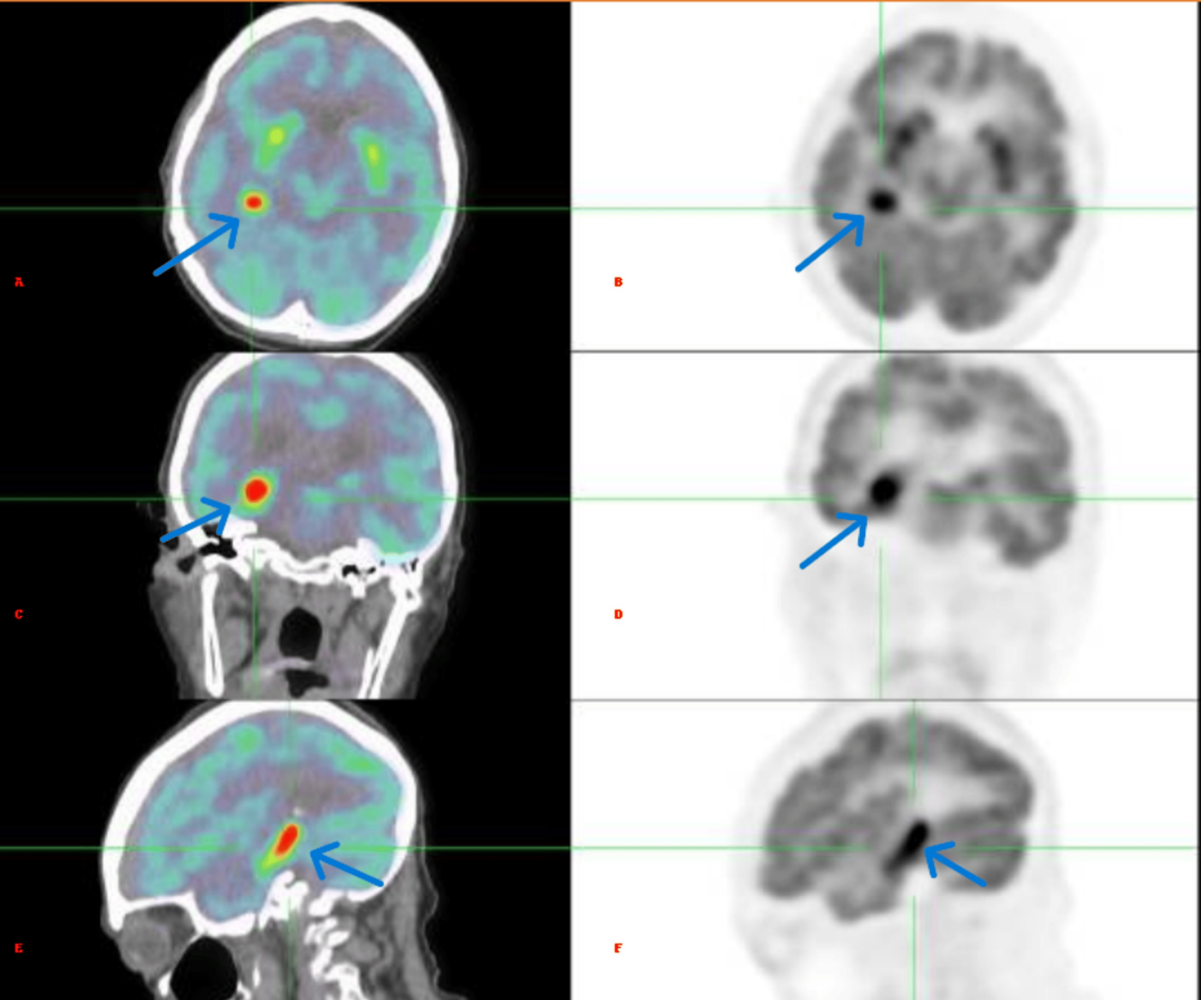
Hypermetabolic temporal lesion (A–C) Fused 18F-FDG PET/CT and (D–F) corresponding PET-only slices in axial, coronal, and sagittal planes, showing a focal hypermetabolic lesion in the right mesial temporal lobe. 18F-FDG PET/CT: 18F-Fluorodeoxyglucose positron emission tomography/computed tomography.
